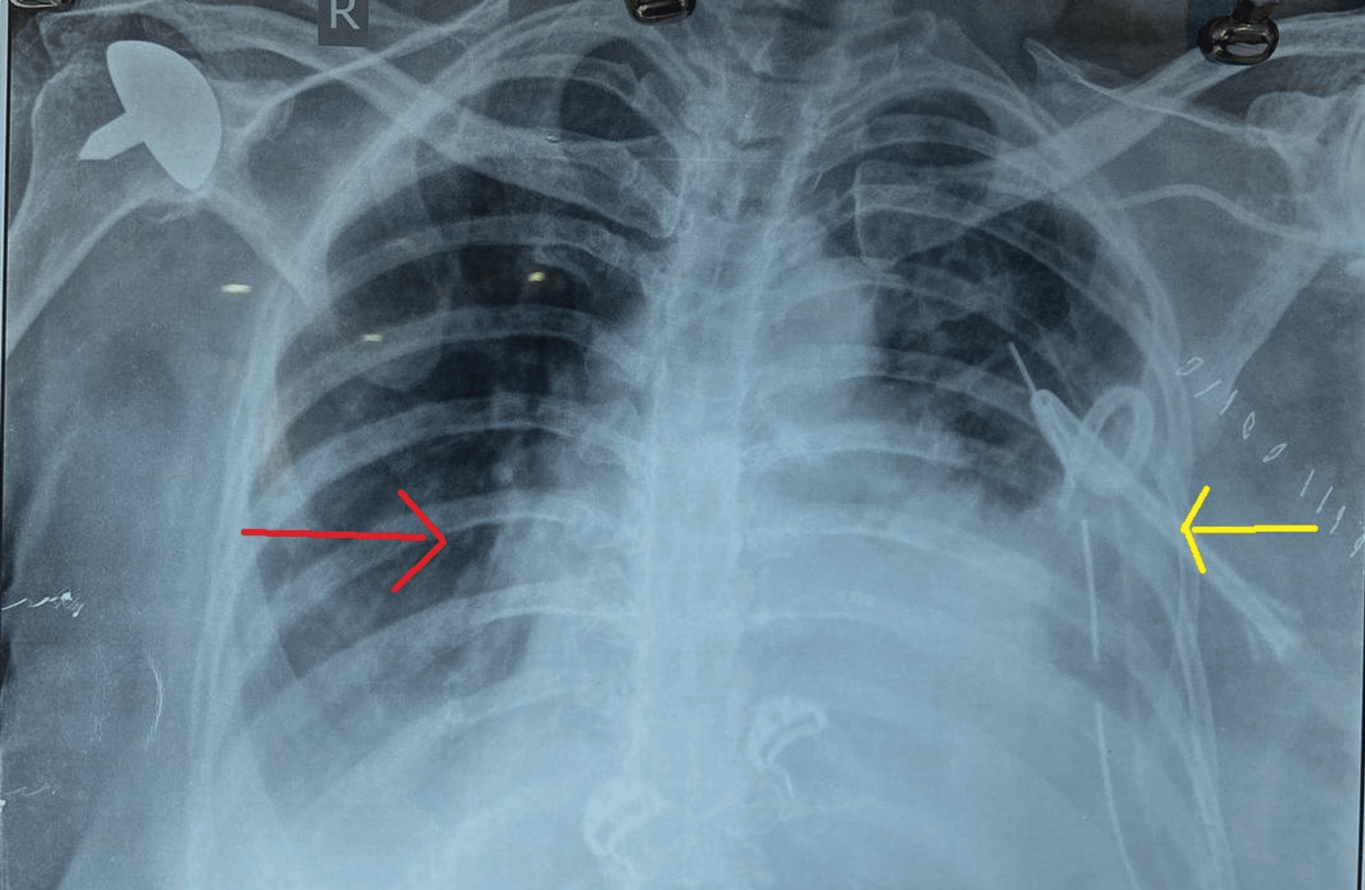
Chest radiograph depicting left-sided pleural effusion (yellow arrow) with subcutaneous emphysema (red arrow)
